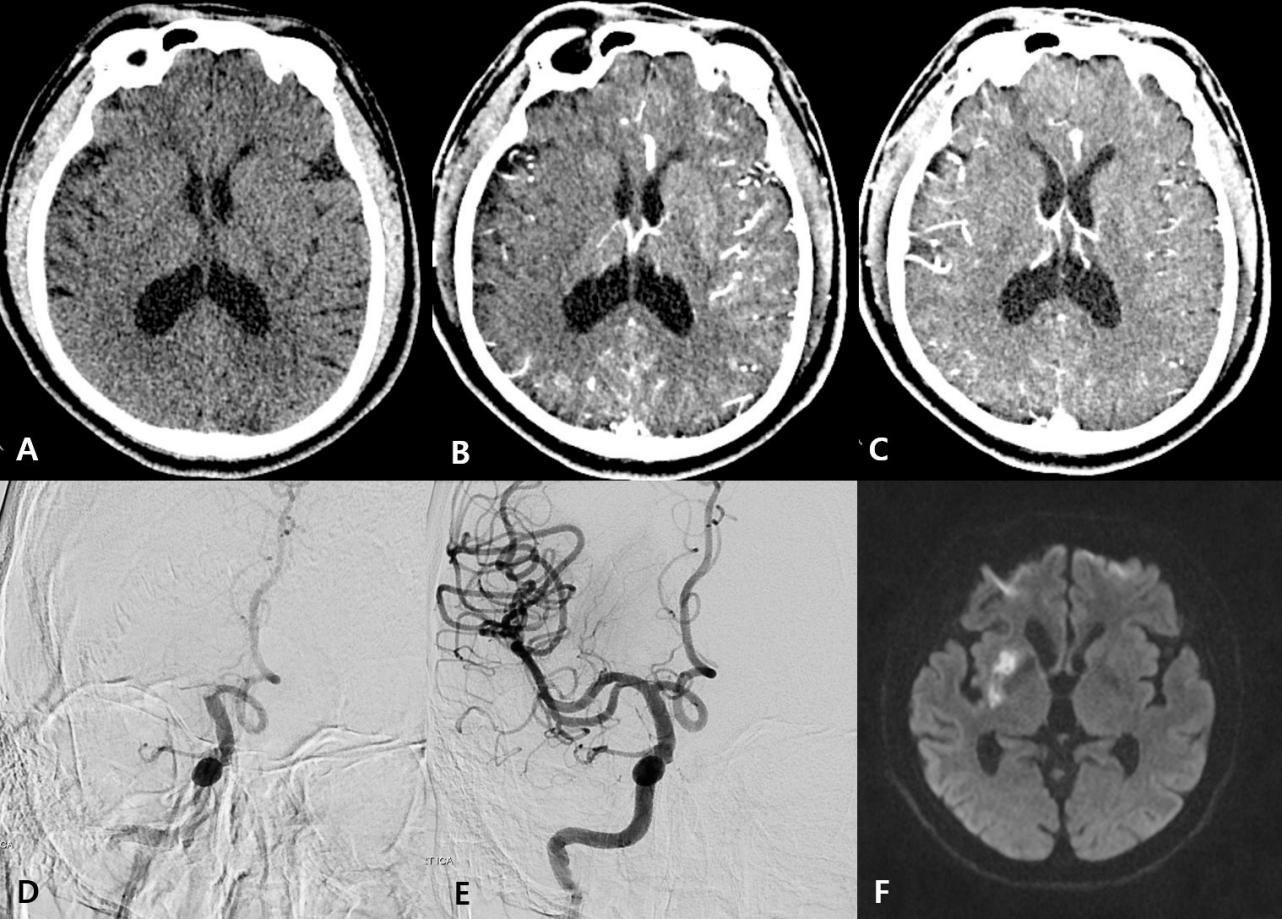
A patient in their 60s’ was admitted with acute left hemiparesis and dysarthria 45 min after symptom onset. (A) Non-contrast computed tomography showed subtle hypodensity of the right insular cortex and lentiform nucleus. (B) Arterial phase computed tomography angiography (CTA) demonstrated hypodensity involving the right insular cortex, lentiform nucleus, internal capsule, and right parietotemporal lobe. (C) Delayed phase CTA demonstrated subtle hypodensity of the right lentiform nucleus. (D) A right carotid angiogram showed an occlusion of the right middle cerebral artery. (E) The angiogram after endovascular thrombectomy showed complete recanalization of the middle cerebral artery. (F) Follow-up diffusion-weighted imaging demonstrated a small area of acute infarction in the right lentiform nucleus.
